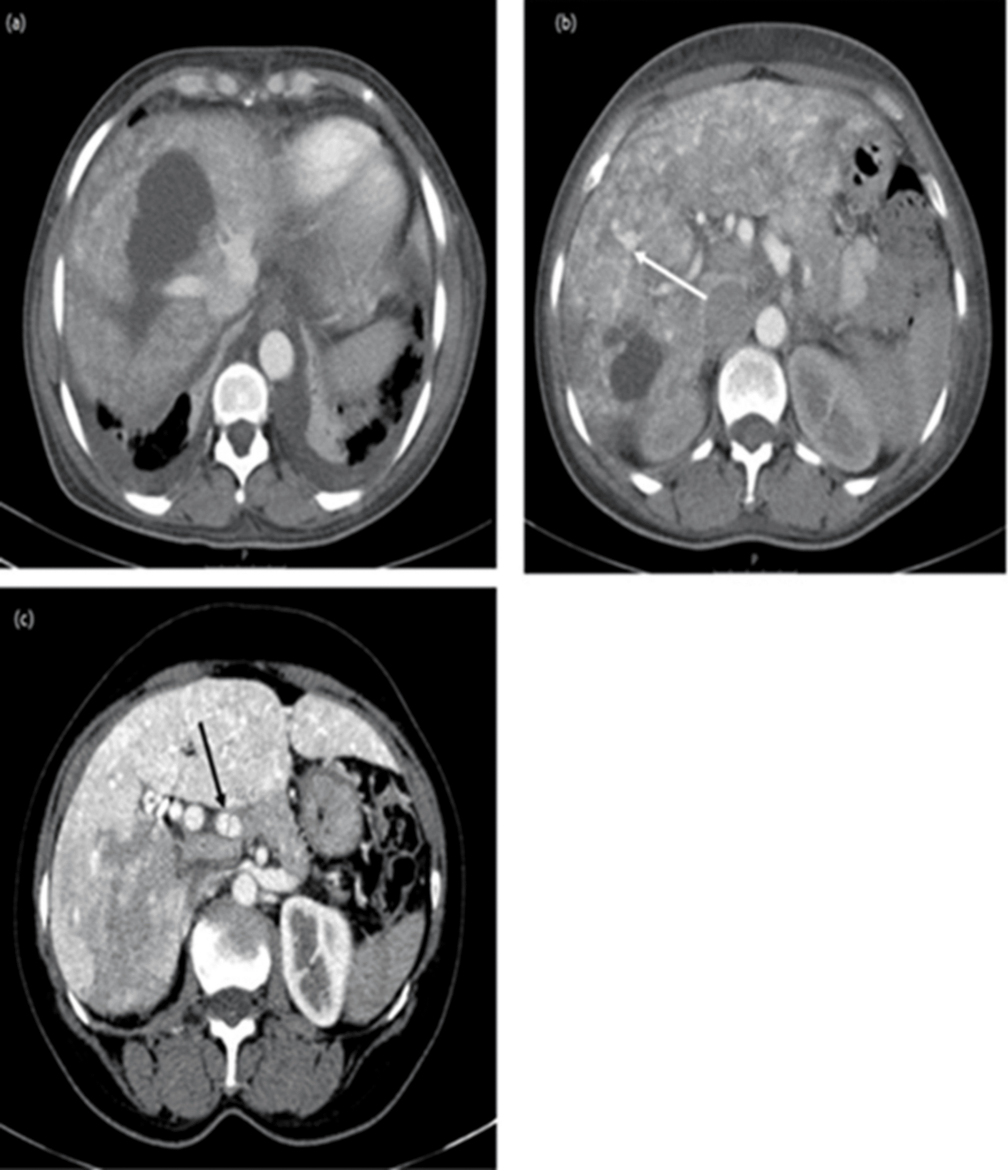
Abdominal CT in arterial phase Liver with signs of chronic liver disease and (b) numerous predominantly peripheraltelangiectasias, as well as arteriovenous shunt or arteriovenous malformations (AVMs) (white arrow). (a) and (b) Hypodense nodular lesions (*), in relation to bile lakes/bilomas. (c) Enlarged hepatic artery (black arrow).
